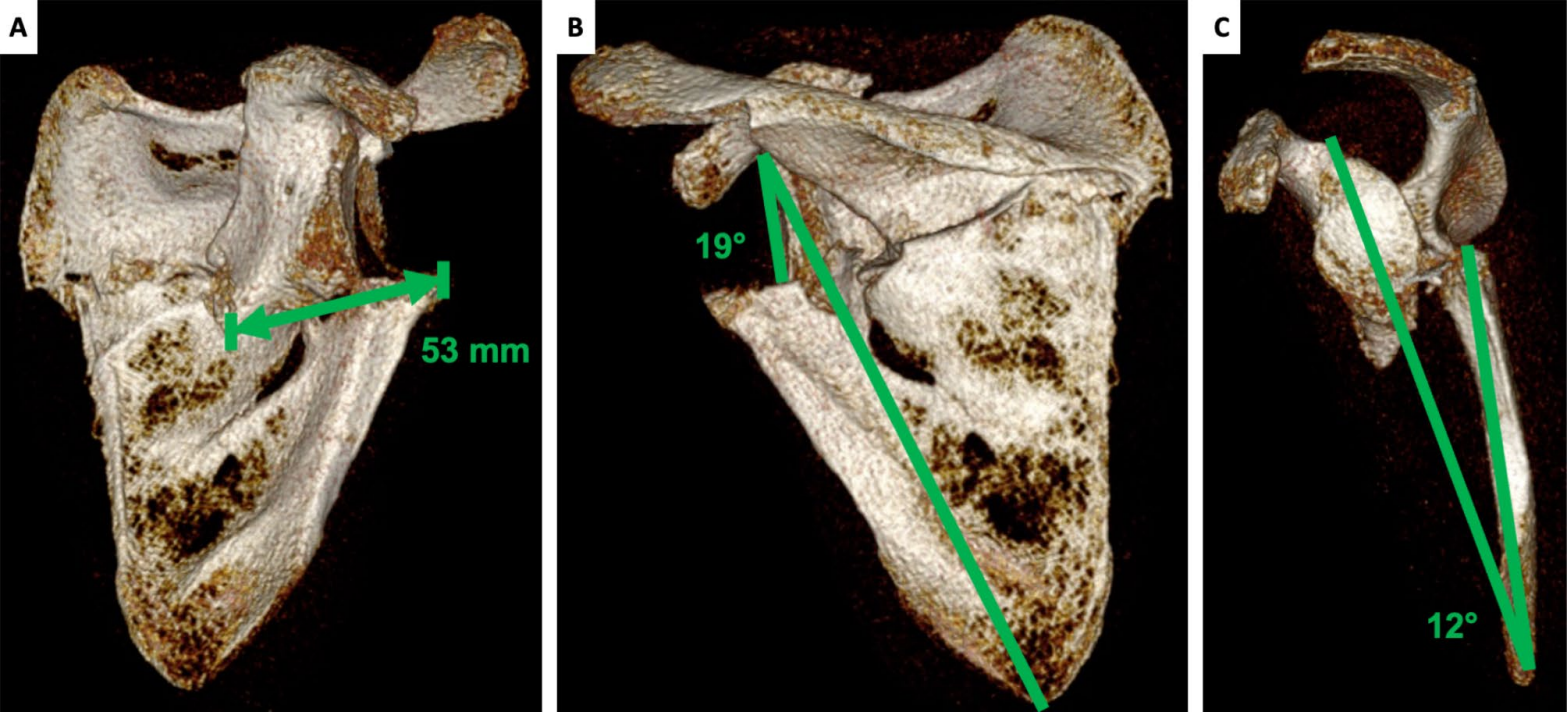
Left scapula deformity values measured on preoperative CT scan. A: lateral border offset 53 mm. B: glenopolar angle 19°. C: angulation deformity 12°
